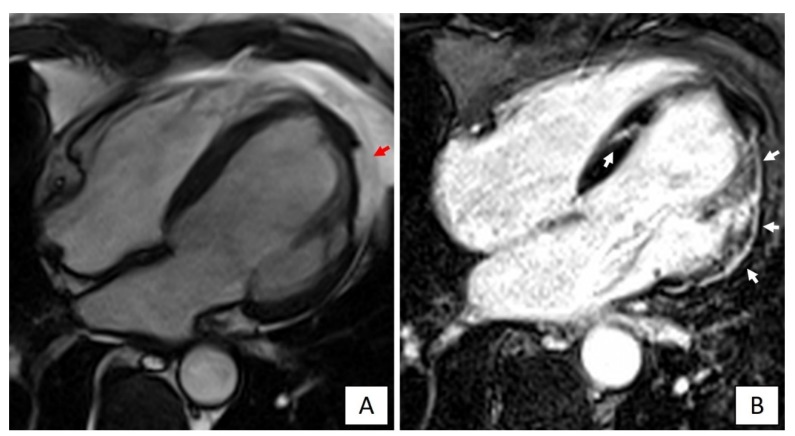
Cardiac magnetic resonance imaging of a patient with *DSP*-related left dominant arrhythmogenic cardiomyopathy. (A) End-diastolic frame of cine cardiac magnetic resonance sequence in long-axis four-chamber view showing fatty infiltration of the lateral wall of the left ventricle (red arrow). (B) Post-contrast image showing myocardial fibrosis in the form of extensive late gadolinium enhancement in the lateral wall and septum of the left ventricle (white arrows).
